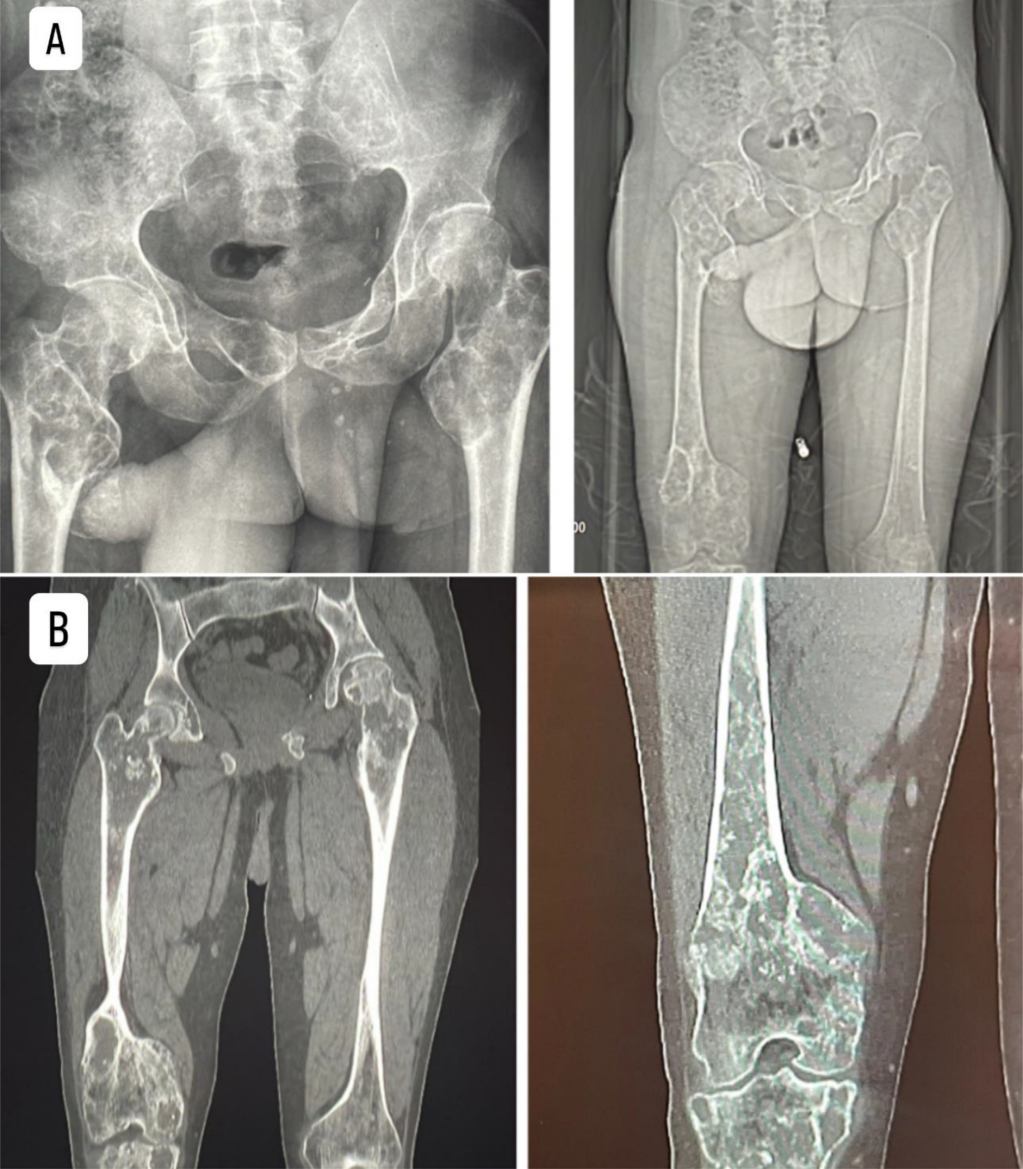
The scout X-ray and pelvic radiograph (A) demonstrate multiple lytic lesions involving the pelvis, proximal femora, iliac bones, and distal femora. These lesions appear expansile and destructive, with cortical thinning and areas of cortical breakthrough, consistent with multiple enchondromas. The bone reconstruction image (B) provides a detailed visualization, confirming the presence of extensive intramedullary lytic lesions in the proximal and distal femur, iliac bones, and pelvic structures. There is significant bone remodeling and cortical destruction.
